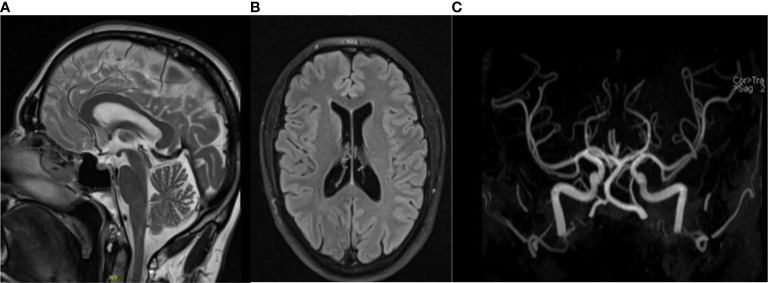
Magnetic resonance imaging depicting T2 flair (A) and coronal (B) and TOF angiography (C) views of the brain, excluding cerebral hemorrhages and stroke.
